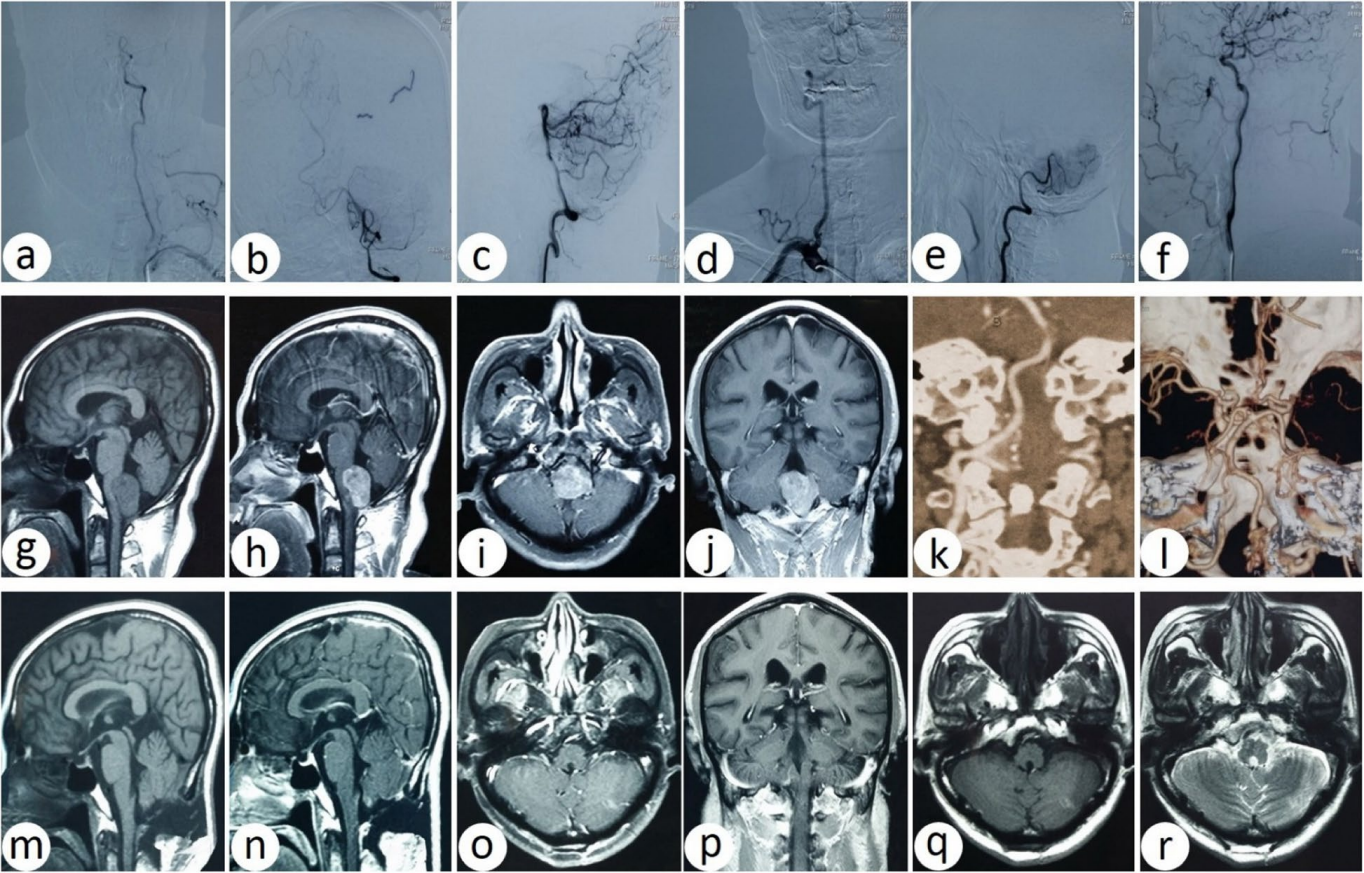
Comparison of preoperative and postoperative images of the foramen magnum meningioma, which is located in the back of the brainstem and superior to the vertebral artery. a–c Orthotopic, oblique, and lateral DSA of the left vertebral artery. d–e Orthotopic and lateral DSA of the right vertebral artery. f Orthotopic DSA of the right cephalic artery. g–h Preoperative sagittal T_1_-weighted MRI and contrast-enhanced MRI. i–j Preoperative axial and coronal T1-weighted contrast-enhanced MRI. k–l Preoperative left vertebral artery 3DCTA image and bilateral vertebral artery image reconstruction. m–n Postoperative sagittal T_1_-weighted and contrast-enhanced MRI. o–p Postoperative axial and coronal T1-weighted contrast-enhanced MRI. q–r Postoperative axial flair and T2-weighted MRI
